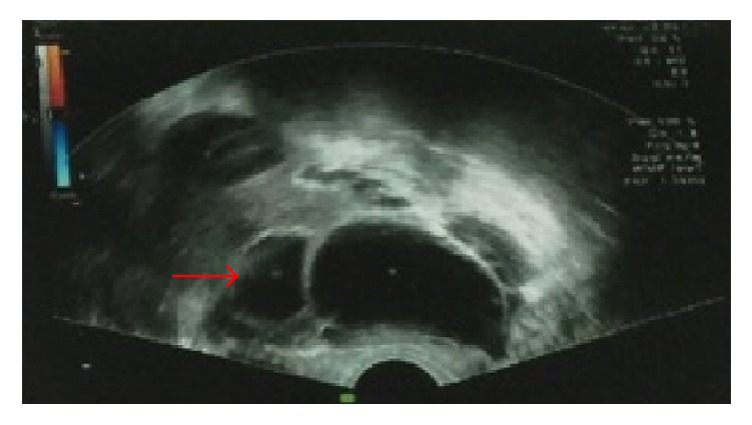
A 29-year-old female patient with primary amenorrhea. Transvaginal ultrasound shows no uterine; red arrow: polycystic ovary tumor with clear boundary.
